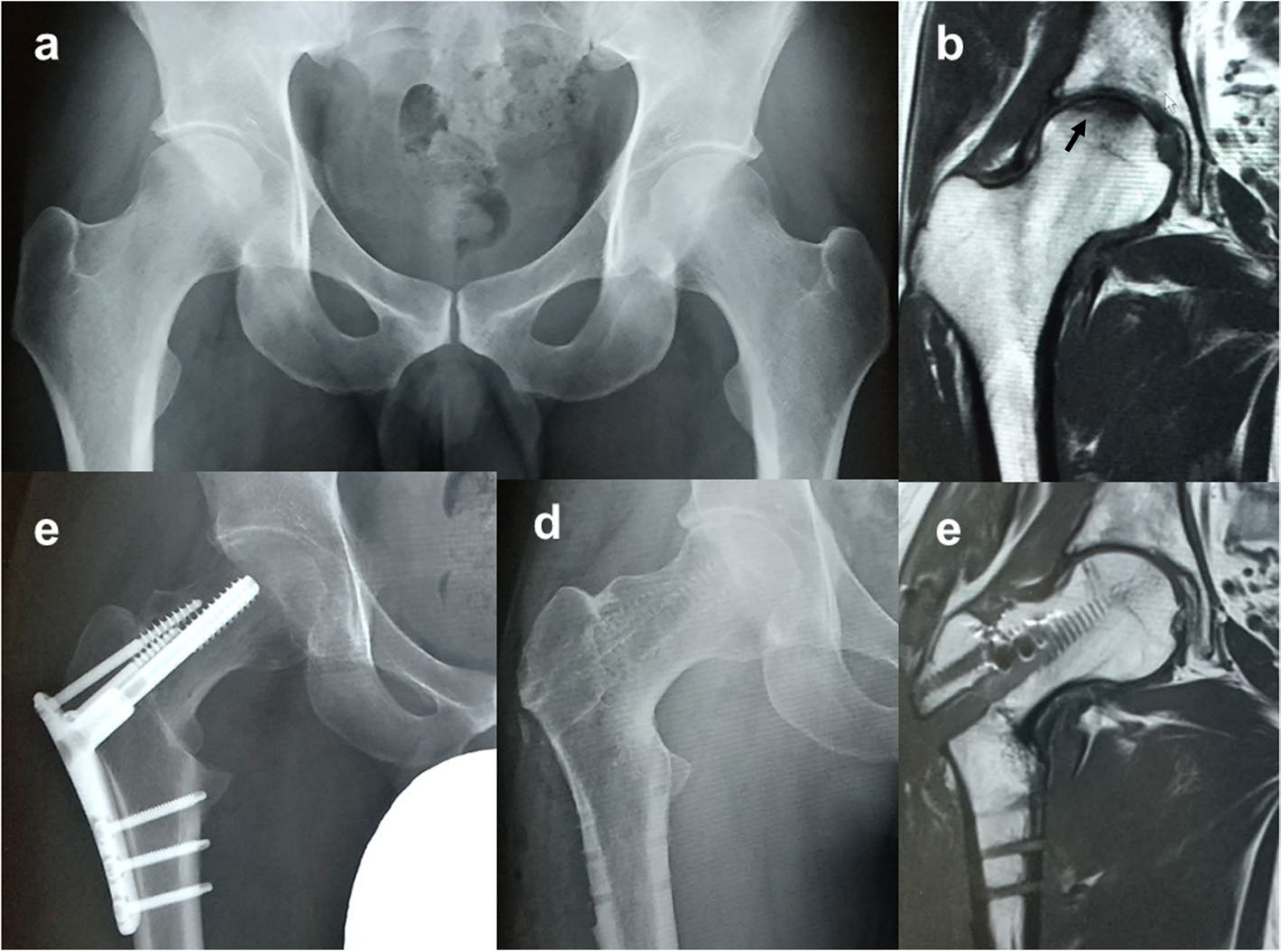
Case 1 (30-year-old man). a An AP radiograph of the right hip at the first visit. b T1-weighted magnetic resonance image (MRI) shows a low-signal area and low-intensity band (arrow) in the weight-bearing region of the right femoral head. c Six months after onset, inter-trochanteric curved varus osteotomy was performed. d AP radiograph obtained 2 years postoperatively showing fusion of the osteotomy site. Although there is slight osteophyte formation, there is no narrowing of the joint space, and hip function is good. e T1-weighted MRI 2 years postoperatively. The low-signal area that was present preoperatively disappeared
